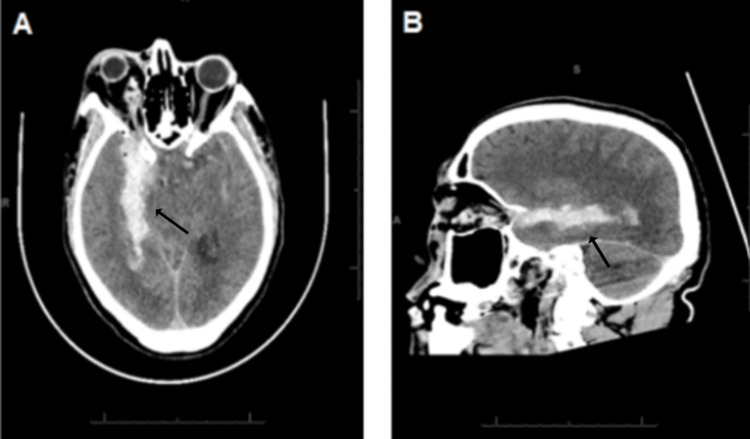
CT of the head after removal of the ballpoint pen. A. Axial CT scan of the head after removal of the pen showing the level of intracranial hemorrhage present.​ B. Sagittal CT scan status post pen removal demonstrated an intraparenchymal and subarachnoid hemorrhage with intraventricular extension along the prior foreign body tract without evidence of hydrocephalus.
